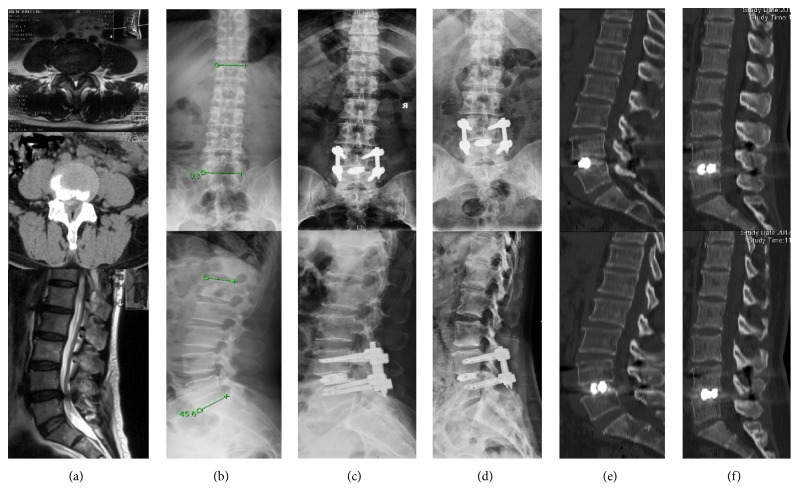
A 57-year-old female patient who had low back pain with right leg pain and numbness for 2 years, intermittent claudication 100m, and was treated by PE-TLIF. (a) Preoperative MRI and CT images showed a moderate lumbar spinal stenosis. (b) Preoperative X-ray image showed no instability. (c) Postoperative X-ray image showed a good implantation position. (d) Six-month follow-up X-ray image showed neither disc space subsidence nor implantation breakage. (e) Postoperative CT scan image. (f) Six-month follow-up CT scan image showed a standard lumbar fusion.
